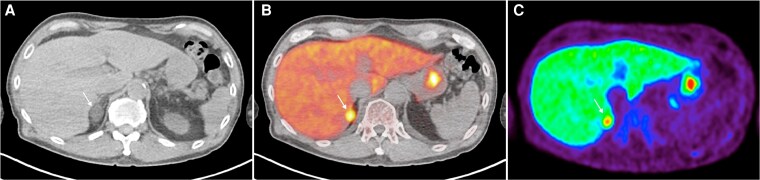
^11^C-MTO PET-CT for case 1—right adrenal nodule (white arrow) seen on CT imaging (A) with a high SUVmax over the right adenoma (32.7) compared to the left adrenal gland (18.7) on the fused PET-CT imaging (B) and PET imaging (C). The SUVmax ratio of right adrenal nodule-to-contralateral gland was 1.75 (greater than cutoff of 1.25 to define lateralization). Abbreviations: ^11^C-MTO PET-CT, ^11^C-metomidate positron emission tomography-computed tomography; SUVmax, maximum standardized uptake value.
